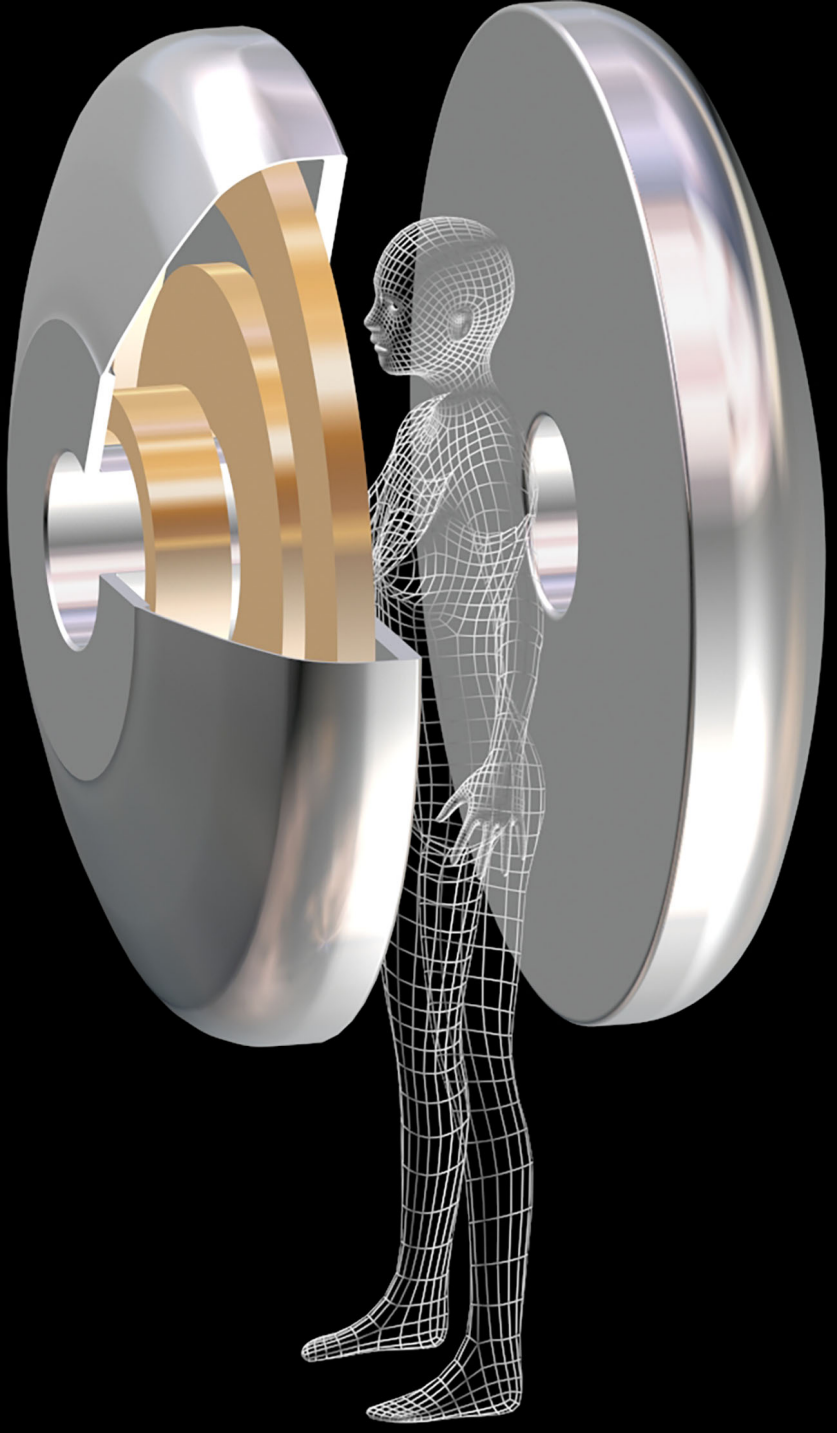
Prototype of a MRI magnet dedicated to breast cancer screening. The superconducting magnet consists in 2 halves. The patient stands between the 2 halves. To reduce size and cost, this magnet can be tailored for scanning one breast at a time (courtesy T. Schild, Irfu/CEA).
